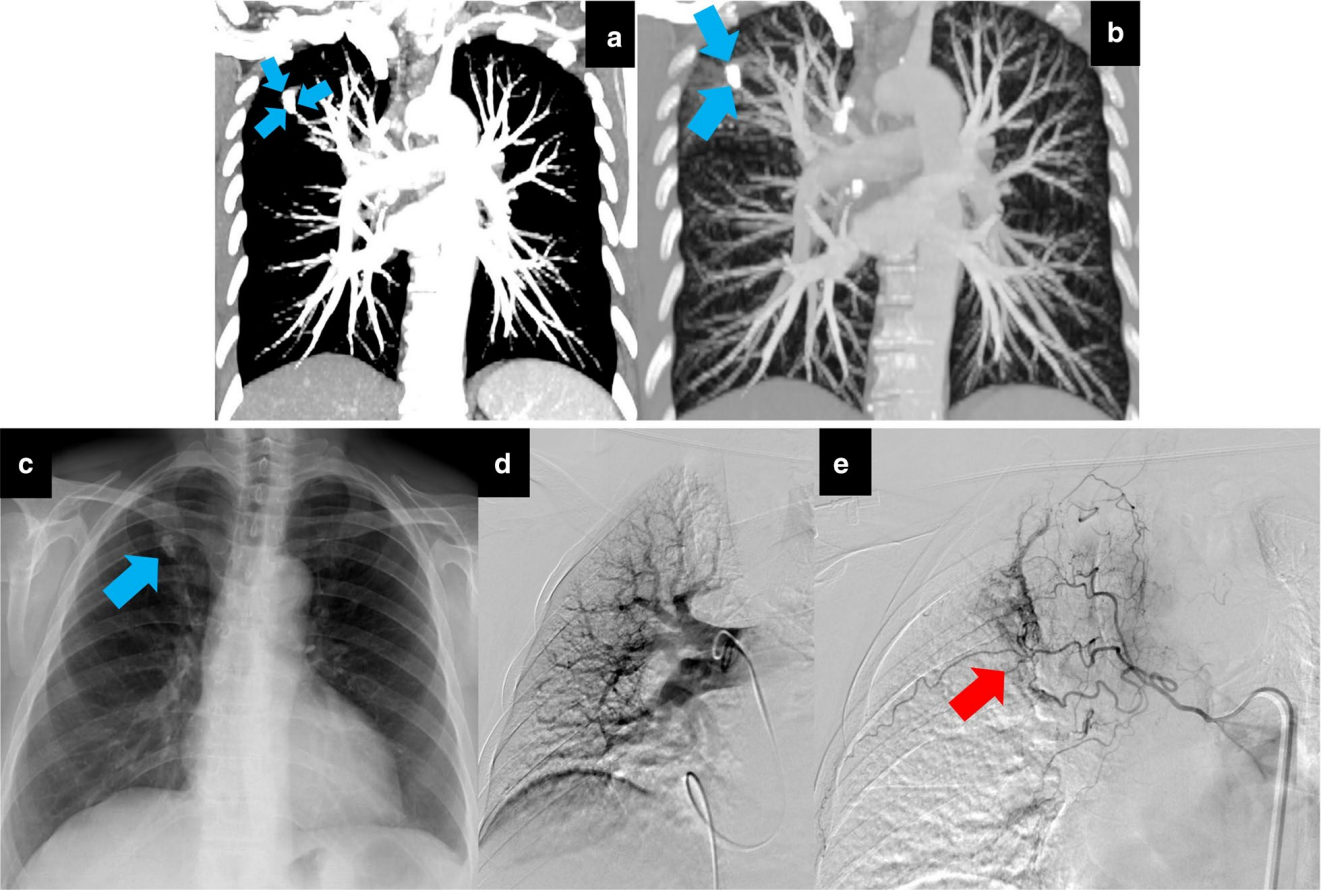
An over-reading (type 1) error with a positive effect on patient management. When evaluating contrast-enhanced CTs, failure to adjust the window settings may cause calcified lesions to be confused with vascular lesions (blue arrows, a, b). A 67-year-old female patient presented with the complaint of hemoptysis to another hospital. Before that admission, she had been investigated several times for hemoptysis in different medical centers via CTA, which had shown no apparent causes and no dilated bronchial artery. The patient had been discharged each time because of negative imaging findings and regression of symptoms. Upon this last admission, pulmonary CTA was performed and reported as «Consistent with pulmonary artery aneurysm in the right lung apex.». The patient was referred urgently to our vascular interventional unit. Invasive pulmonary angiography revealed nothing abnormal (d). It was later understood that a calcified nodule at the right apex (blue arrow, c) caused the over-reading error by mimicking an aneurysm. It was decided to perform bronchial arteriography following pulmonary angiography while the patient was already in the operating room because of the long-term recurrent hemoptysis history. Dilated, distorted and tortuous bronchial vessels detected on bronchial arteriography (red arrow, e) were embolized. Throughout a one-year follow-up period, the patient had no recurrence of hemoptysis. As shown in this case, errors do not always cause patient harm. Occasionally, an error may have a positive effect on patient management. The diagnosis and treatment process of the underlying cause of the patient’s recurrent hemoptysis was accelerated owing to an over-reading error causing an urgent referral to our angiography unit
